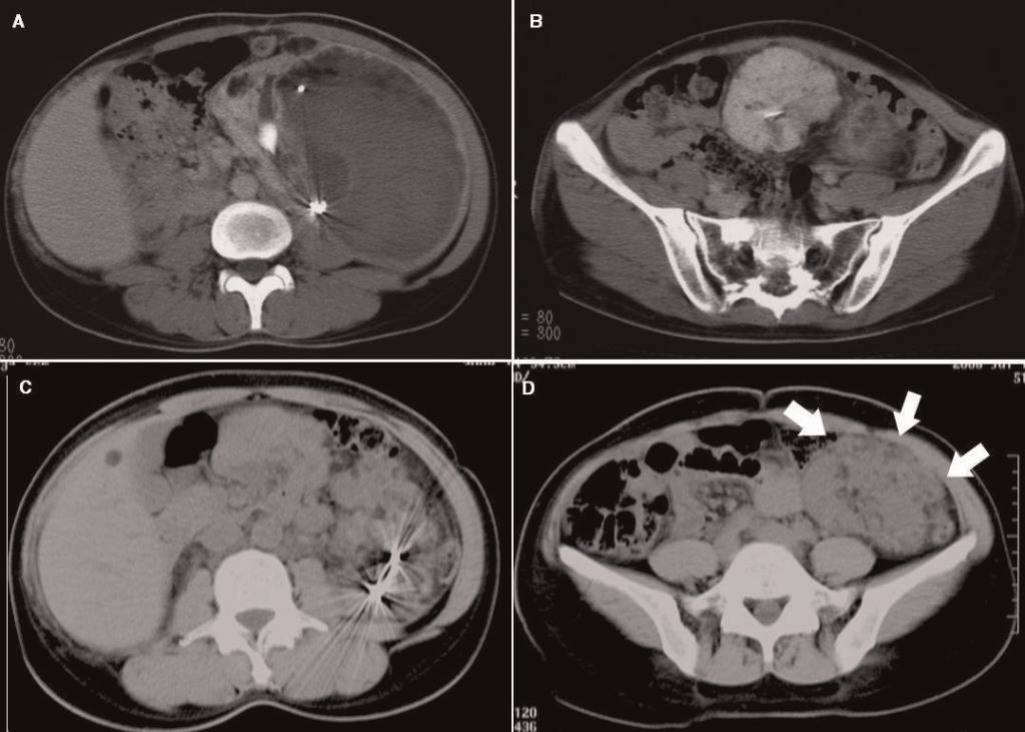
CT scans at half a year (A-B) and 5 years after (C-D) the treatment (A-D) Gradual diminution of the retroperitoneal hematoma and angiomuscular components of the tumor. The conserved renal parenchyma is satisfactorily visualized. (C-D) CT performed 5 years after the treatment demonstrated disappearance of the hematoma and angiomuscular components, but enlargement of the angiomyolipoma developing from the conserved kidney (thick arrows).
